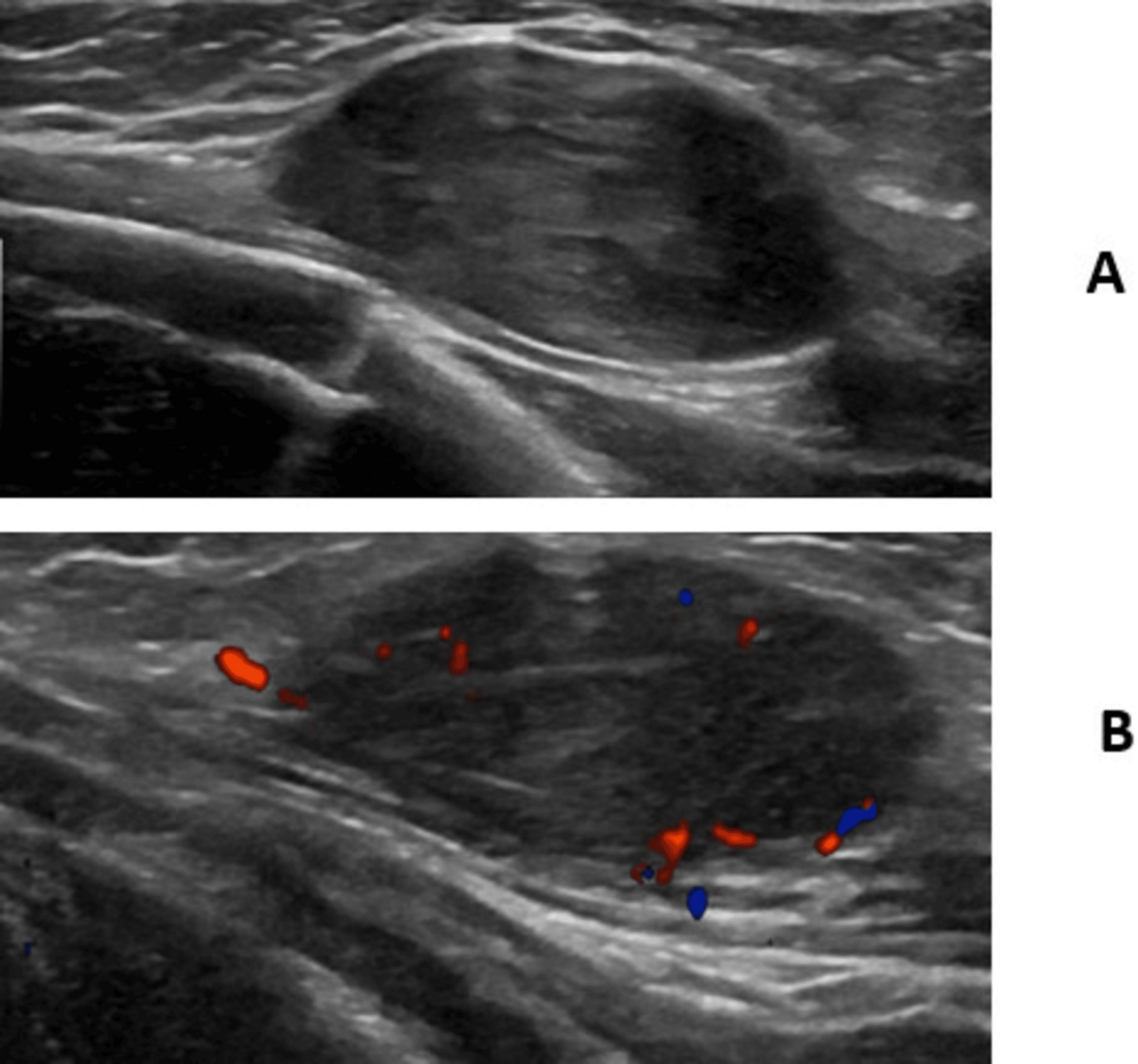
Grayscale (A) and Doppler (B) images of a palpable mass in the left anterior chest inferior to the clavicle. There is minimal vascularity.
